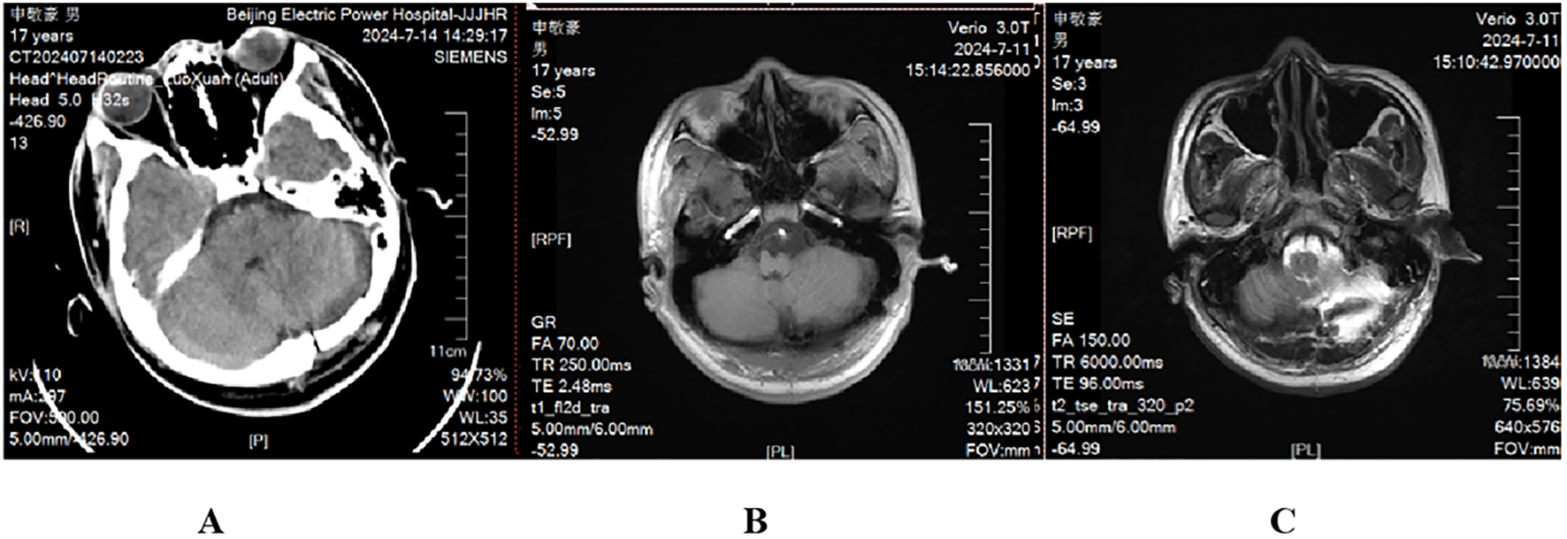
Postoperative CT and MRI results. CT scanning (A) and T1-weighted (B) and T2-weighted (C) scanning showed that the lesion was completely resected.
